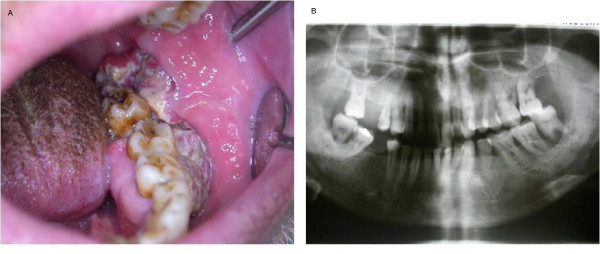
(a) Intra-oral view showing the clinical aspect of case 1. (b) Panoramic radiograph showing bone loss in the left mandibular third molar region of case 1.
